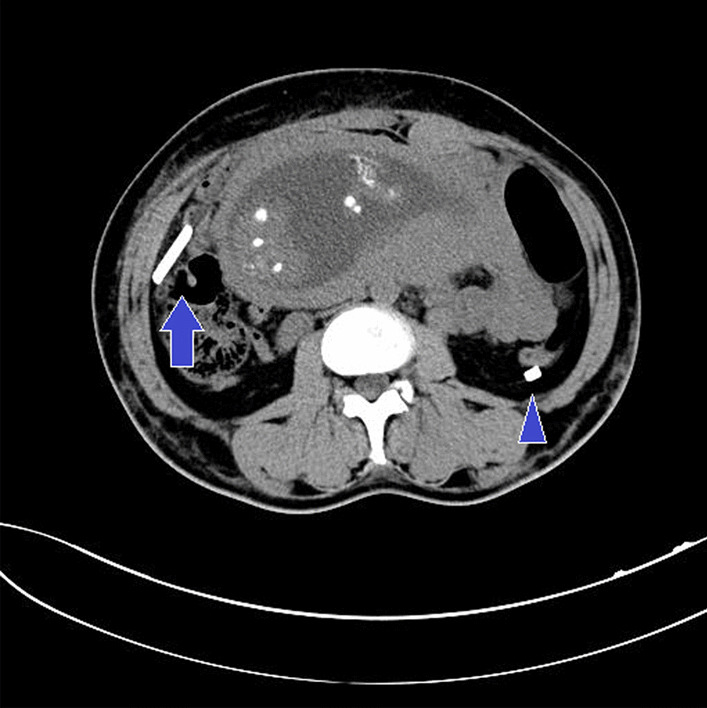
Upper abdominal plain axial CT at 22 weeks gestation. Two hyperdense foci believed to be glass shards are present in the right hypochondrium (arrow) and near the dorsal side of the descending colon (arrowhead). As the patient was pregnant, the plastic surgeon restricted the scanned area to the upper abdomen
